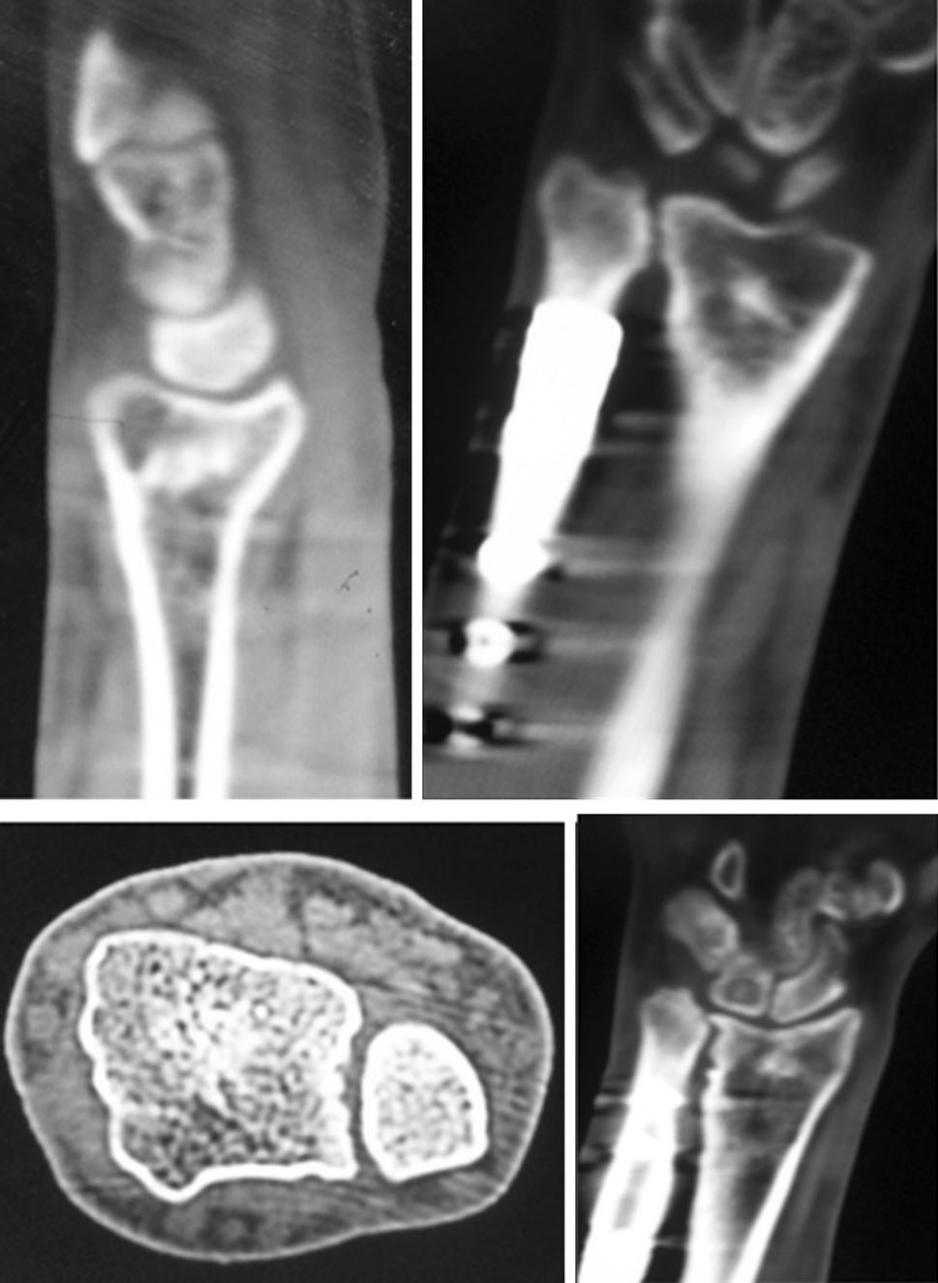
CT scan of wrist of same patient at 2 years
